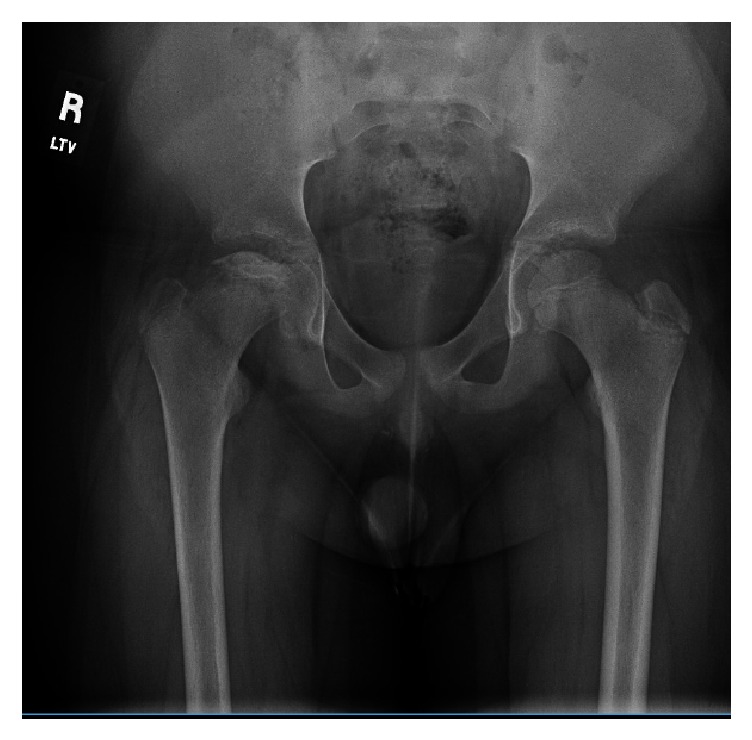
AP pelvis X-ray obtained 12 weeks from initial presentation with progressive flattening of femoral epiphysis indicative of segmental collapse seen in non-Perthes osteonecrosis.
